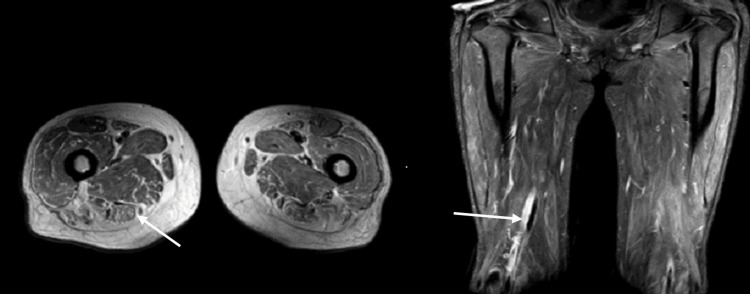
MRI of the thigh showed high T2/STIR signals throughout the musculature of the thigh and a visualized pelvis suggestive of myositis. (A) Transverse plane; (B) Coronal plane MRI: magnetic resonance imaging; STIR: short inversion time inversion recovery
